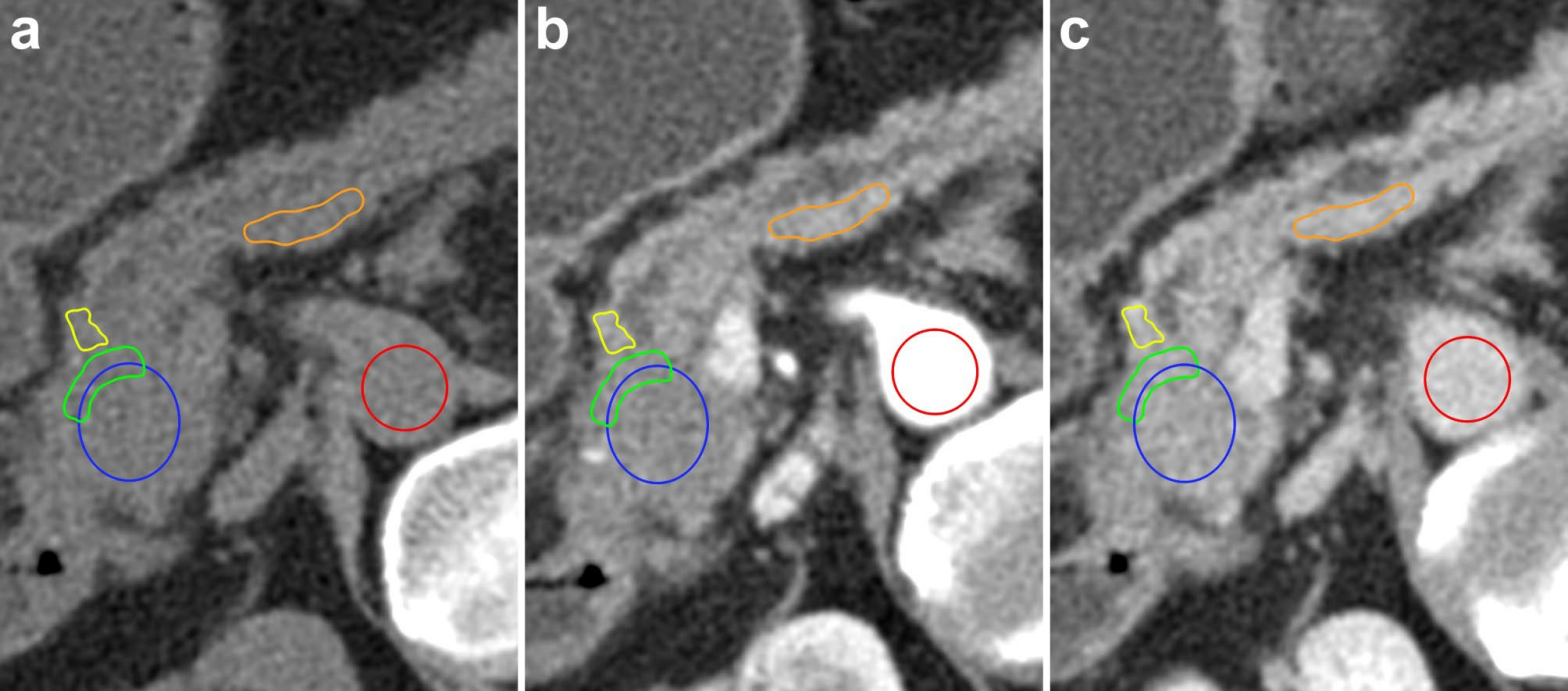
Placement of regions of interest (ROIs). Shown are the ROIs in a patient with a PDAC in the pancreatic head in the (a) non-contrast, (b) late arterial, and (c) portal venous phase; CT acquired in an oblique, 30°, right-sided down position^20^. Blue ROI *tumor*: oval/round, centered in the middle of the tumor, with a margin of at least 2 mm to the border of the tumor. Green ROI *tumor periphery*: freehand, approximately 5 mm thick, peripheral tumor part. Yellow ROI *upstream parenchyma—border to tumor*: freehand, upstream parenchyma directly adjacent to the tumor. Orange ROI *upstream parenchyma—best measurable:* oval or freehand, best measurable area of upstream non-neoplastic tissue. Red ROI *aorta*: oval/round, in the aortic lumen, diameter ~ 15 mm.
